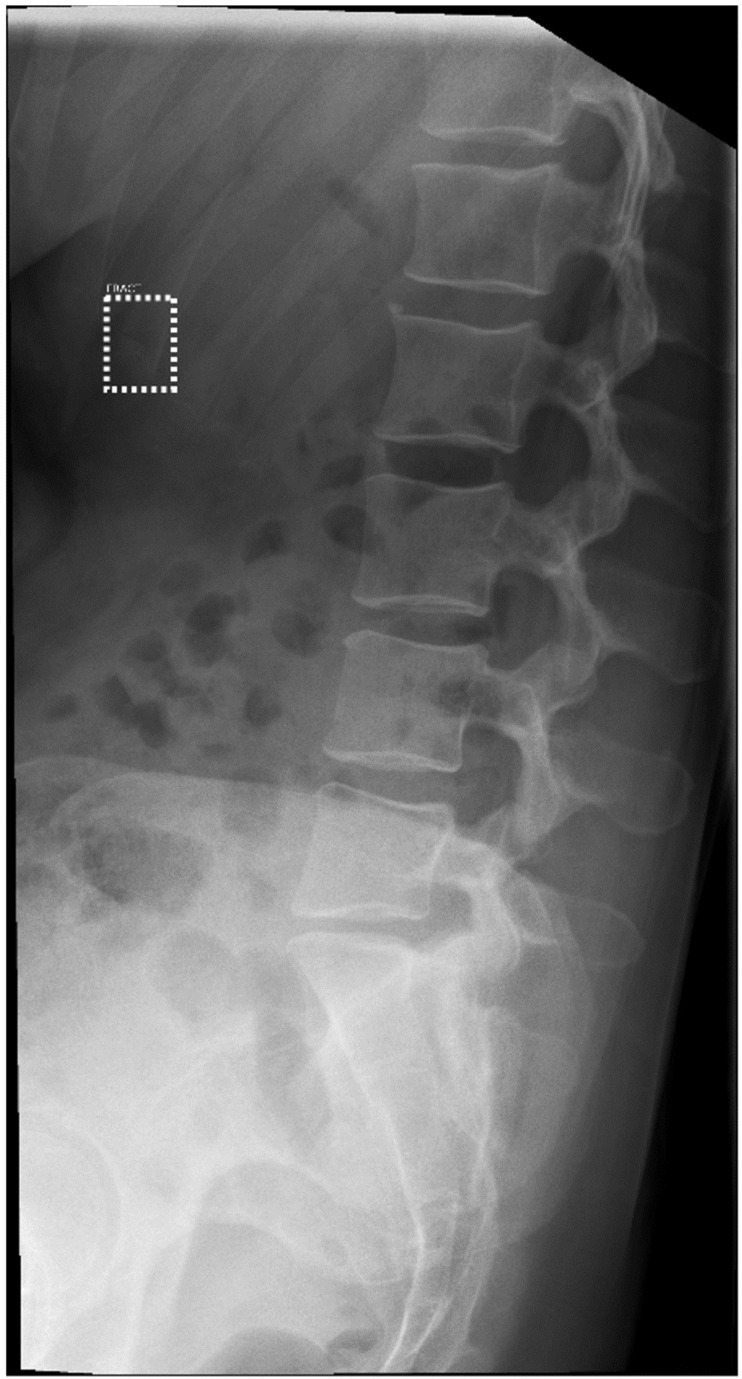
Lateral radiograph of the lumbar spine. In multiple such images, the AI incorrectly marks rib overlay as a possible fracture, noted here by the dashed bounding box marking a “Doubt” fracture.
